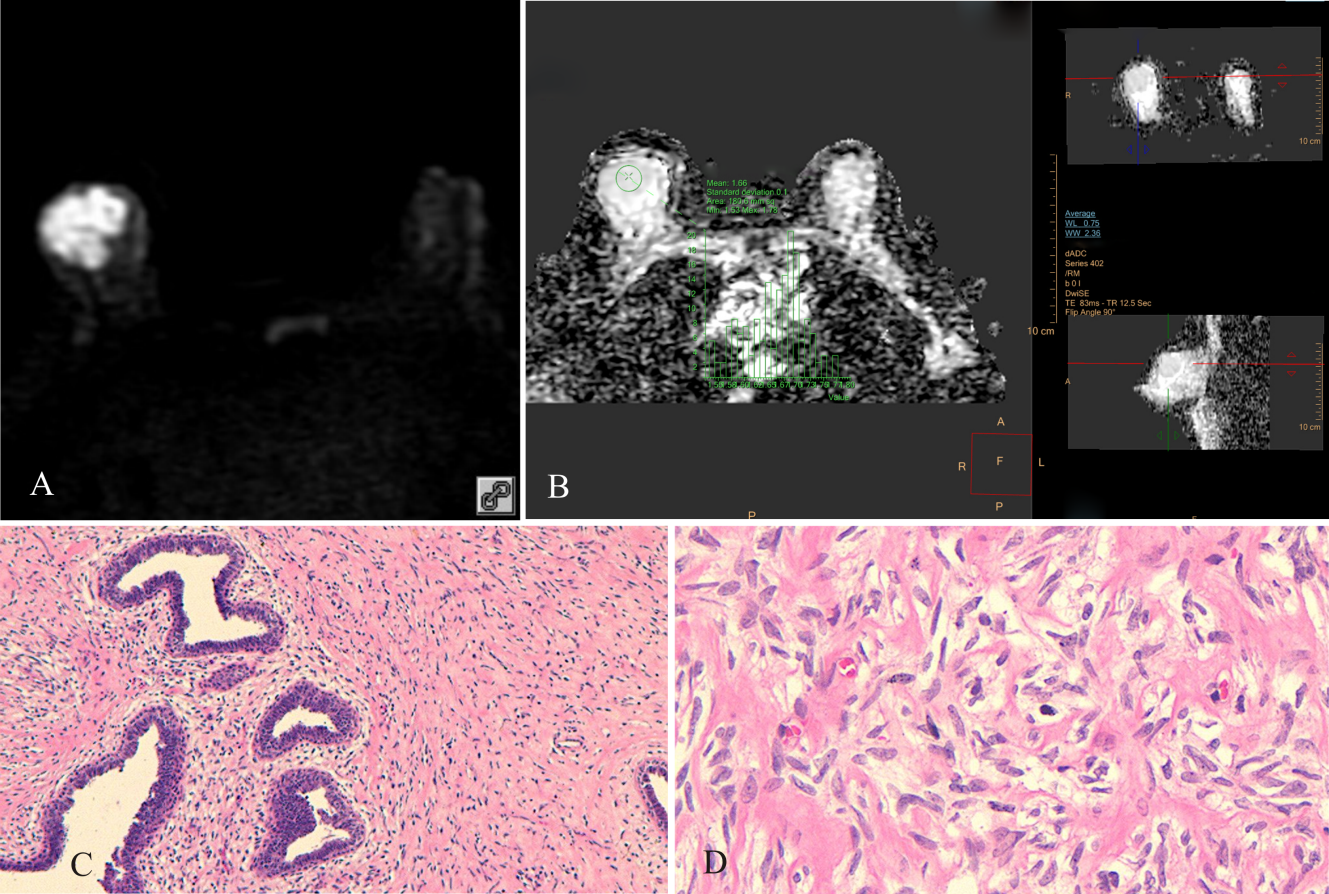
Borderline PT of the right breast, female, age 45 years. (A) DWI (b800 s/mm^2^): mixed hyperintense signal; (B) ADC map: isointense signal, ADC mean = 1.66×10^−3^ mm^2^/s; (C) HE ×100: uneven distribution of tumor stromal cells, high cell density in some areas, low cell density in other areas; (D) HE ×400: tumor stromal cells had “tadpole-like” nuclei, cells were closely packed.
